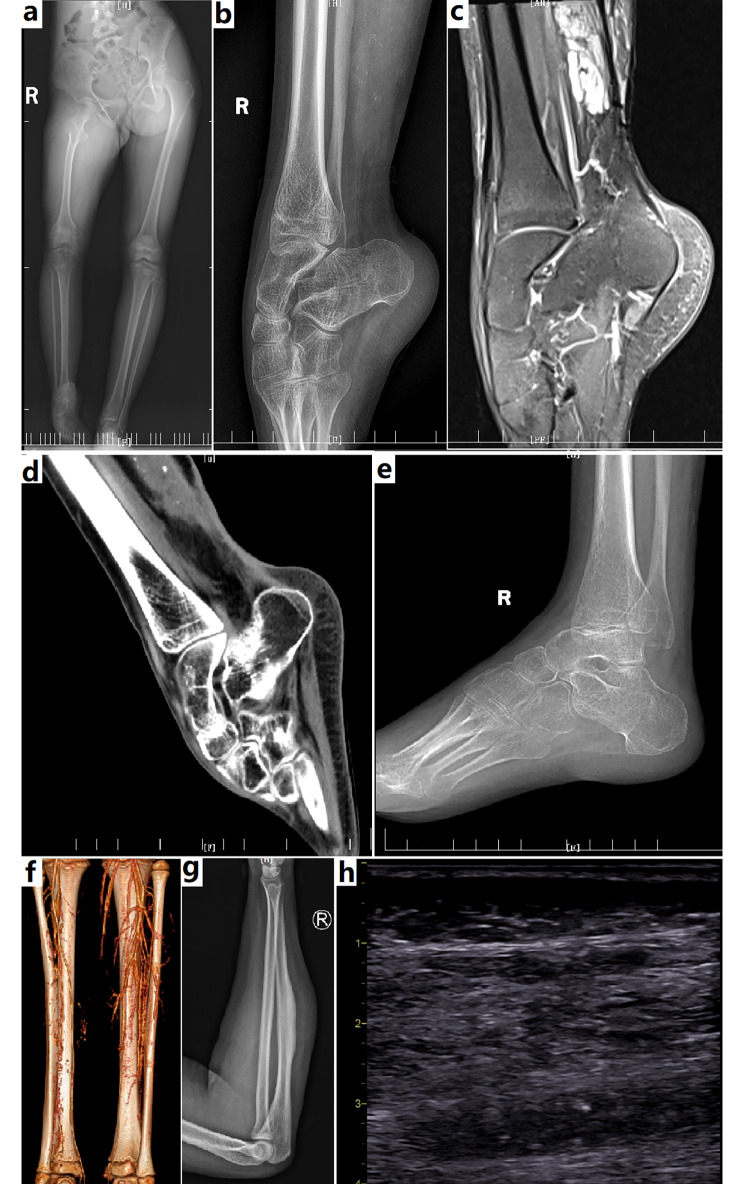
Imaging findings of FAVA. In a patient with FAVA of calf, a, X-ray plain radiography demonstrated pelvic tilt, leg length discrepancy, and fibular deformity. b, Ankle deformity with ankylosis, and pes cavus was noted on X-ray plain radiography. Intralesional foci of calcification or metaplastic bone tissue could also be seen. c, MRI revealed a mass with heterogeneous high signal infiltrating the posterior muscles in calf. d, CT scan demonstrated the mass contained infiltrative low density tissue, indicating a fatty component. e, She re-walked following radical resection, Achilles tenotomy, and relaxation of ankle capsule. f, In a case of FAVA in calf, contrast-enhanced CT scan revealed decreased arterial branches, and ectatic veins in the affected limb. Note the fibular deformity and leg atrophy. g, X-ray plain radiography revealed thickened cortex of the ulna in a case with FAVA of cubitalis posterior. h, FAVA manifested as a solid, noncompressible, and heterogeneous echogenic mass entirely replacing the normal fibrillary pattern within the affected muscle on ultrasonography study
